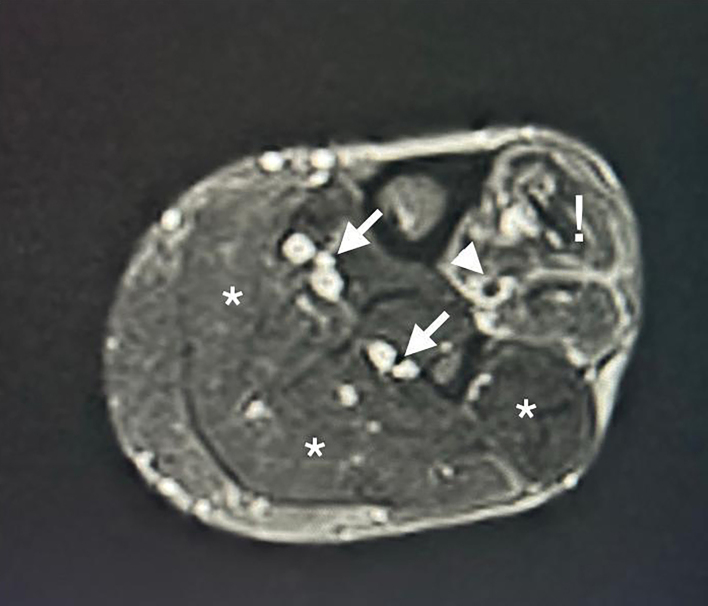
Magnetic resonance imaging of the leg with intravenous contrast, transverse slice: the arrows indicate the posterior tibial and fibular arteries, with flow maintained. The arrowhead indicates the anterior tibial artery, with flow absent. The exclamation mark corresponds to the musculature of the anterior compartment. The asterisks correspond to the musculature of other compartments.
